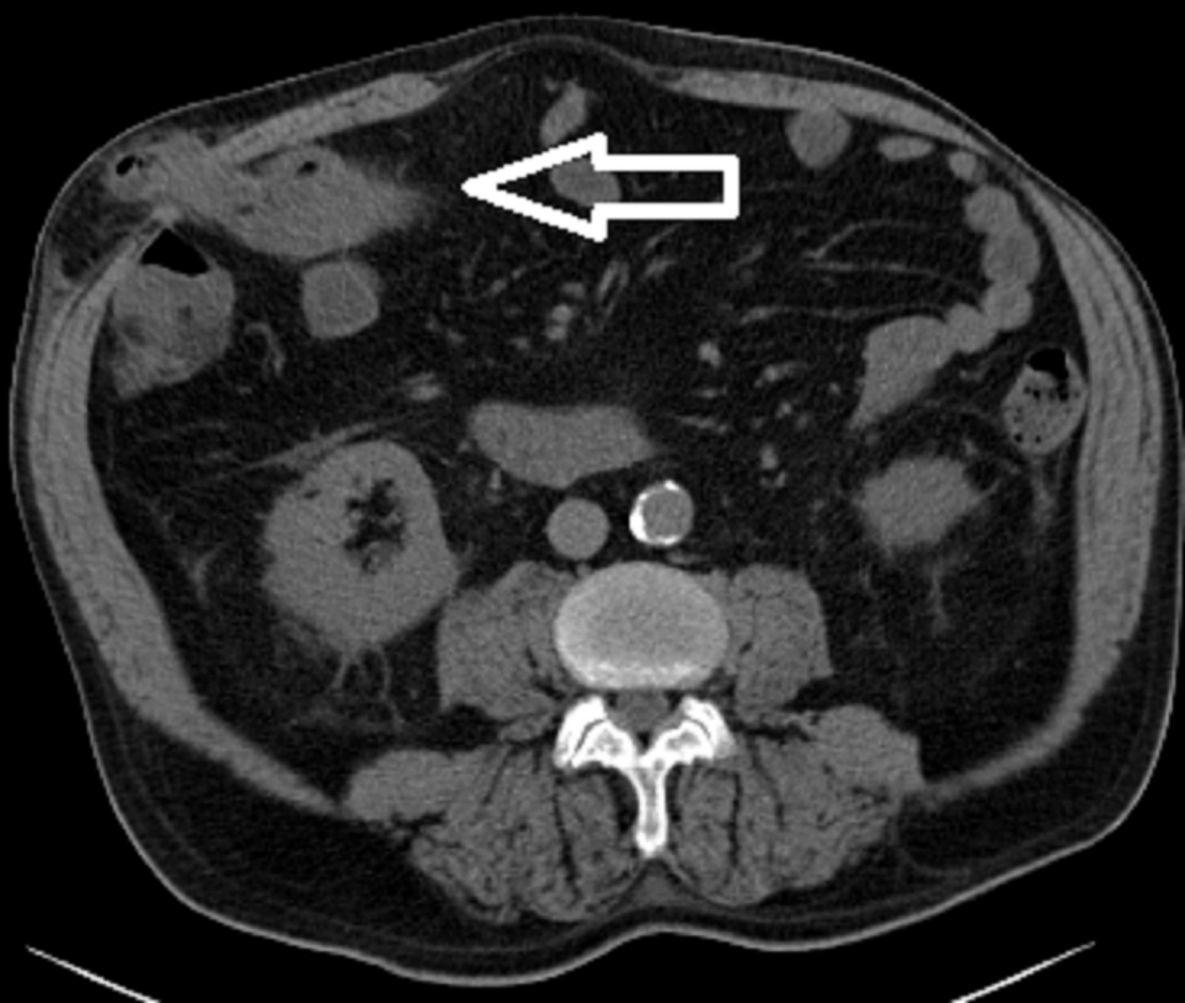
Abdominal wall Spigelian hernia. Computed tomography (CT) scan abdomen showing colonic extension from the hepatic flexure back inferolaterally into a Spigelian hernia with mild fat stranding. The white arrow shows the hernia penetrating through the abdominal wall.
